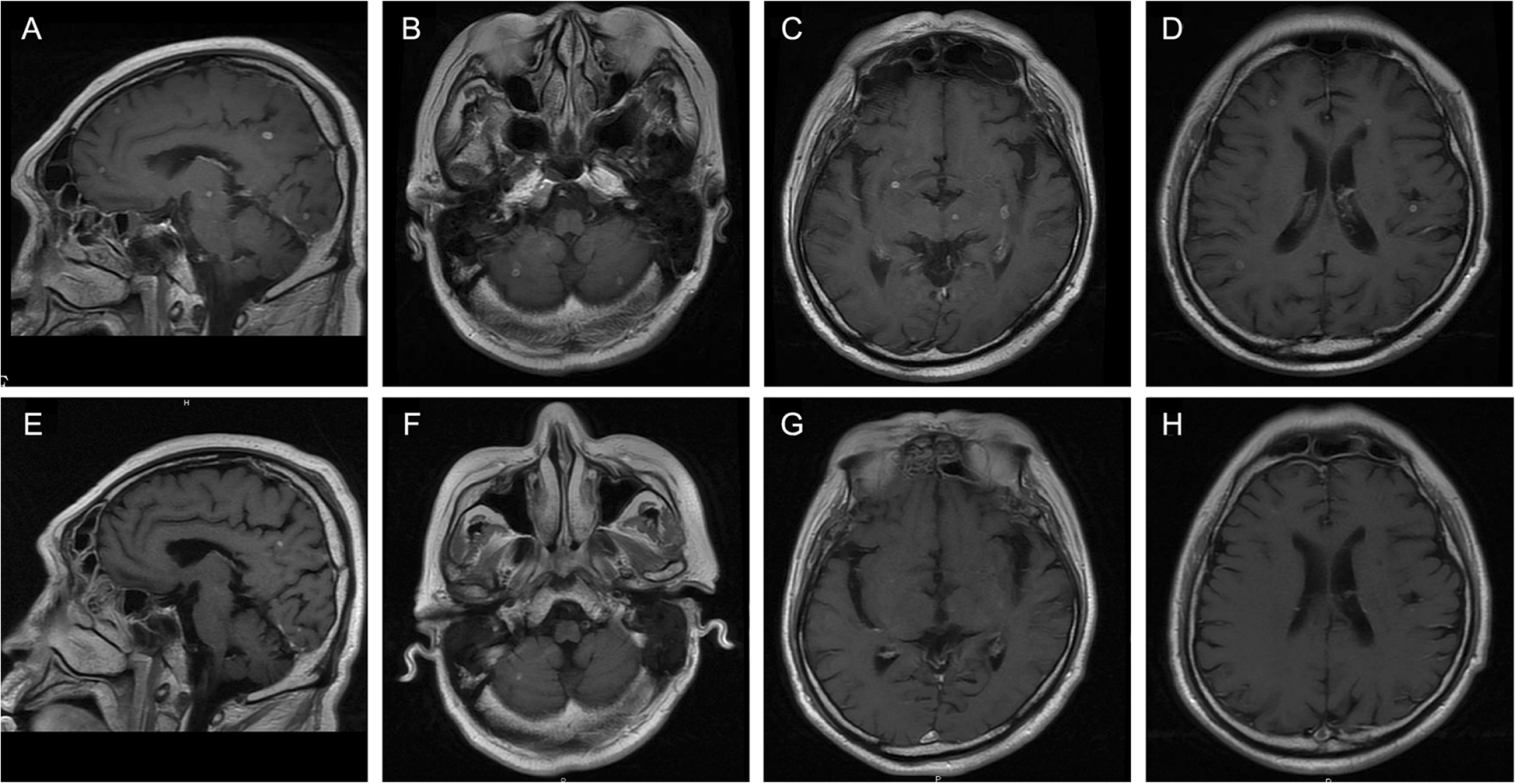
Magnetic resonance imaging of the brain. Gadolinium contrast T1 images, with sagittal reformat (a) and axial reformat (b, c, d) images of the brain showing ring-enhancing lesions in the bilateral brain and cerebellum. e-h: Reviewed MRI scan of the brain showing that some lesions had decreased and some had disappeared after one and half months of SMZ treatment
